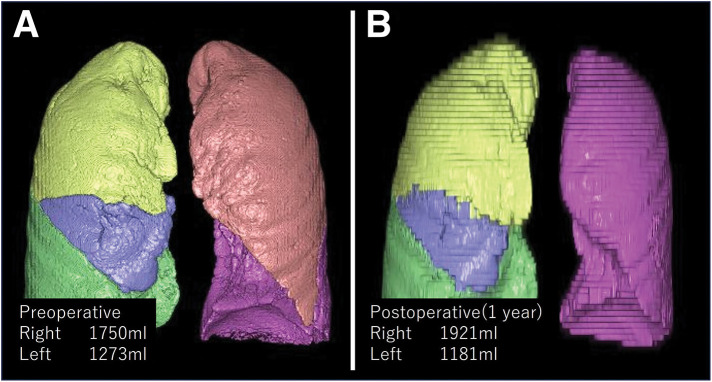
(A) Preoperative 3D-CT volumetry. The right and left lung volumes were 1750 and 1273 mL, respectively. (B) Postoperative 3D-CT volumetry at 1 year after left upper lobectomy. The total left lung volume decreased to 1181 mL, but the remaining left lower lobe demonstrated significant compensatory hyperinflation, expanding into the space of the resected lobe. The contralateral right lung also showed an increased total volume of 1921 mL.
